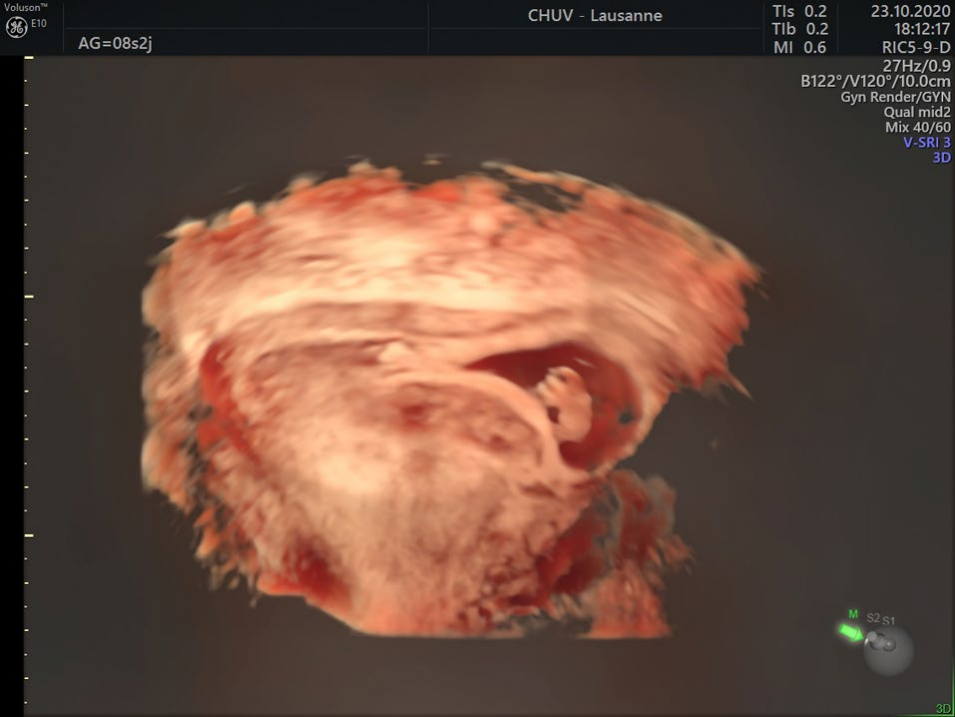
3D Ultrasound coronal view of angular pregnancy at 8wg
